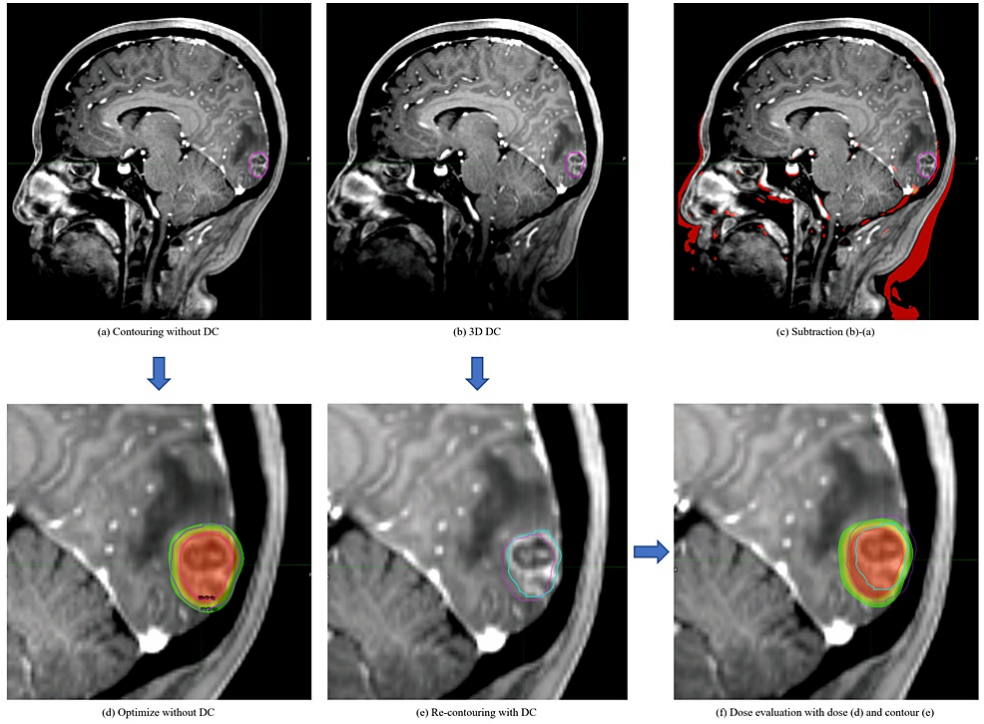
Dose distribution comparison between tumor delineation with and without distortion correction (DC) (a) GTV on MRI without DC; (b) How GTV contoured on MRI without DC (pink line) looks like on MRI with 3D-DC. GTV is not covering some parts, especially the outer part, of the contrast-enhanced tumor; (c) Geometric difference between (a) and (b). The gratitude of the distortion depends on the distance from the central axis; (d) A stereotactic radiotherapy plan optimized based on MRI without DC showing beautiful coverage of the GTV and PTV; (e) Re-contouring performed on MRI with DC (blue). 2-3 mm difference is captured; (f) Actual dose distribution the patient will receive if the plan was made on MRI without DC. PTV coverage and dose to GTV are both inadequate.
